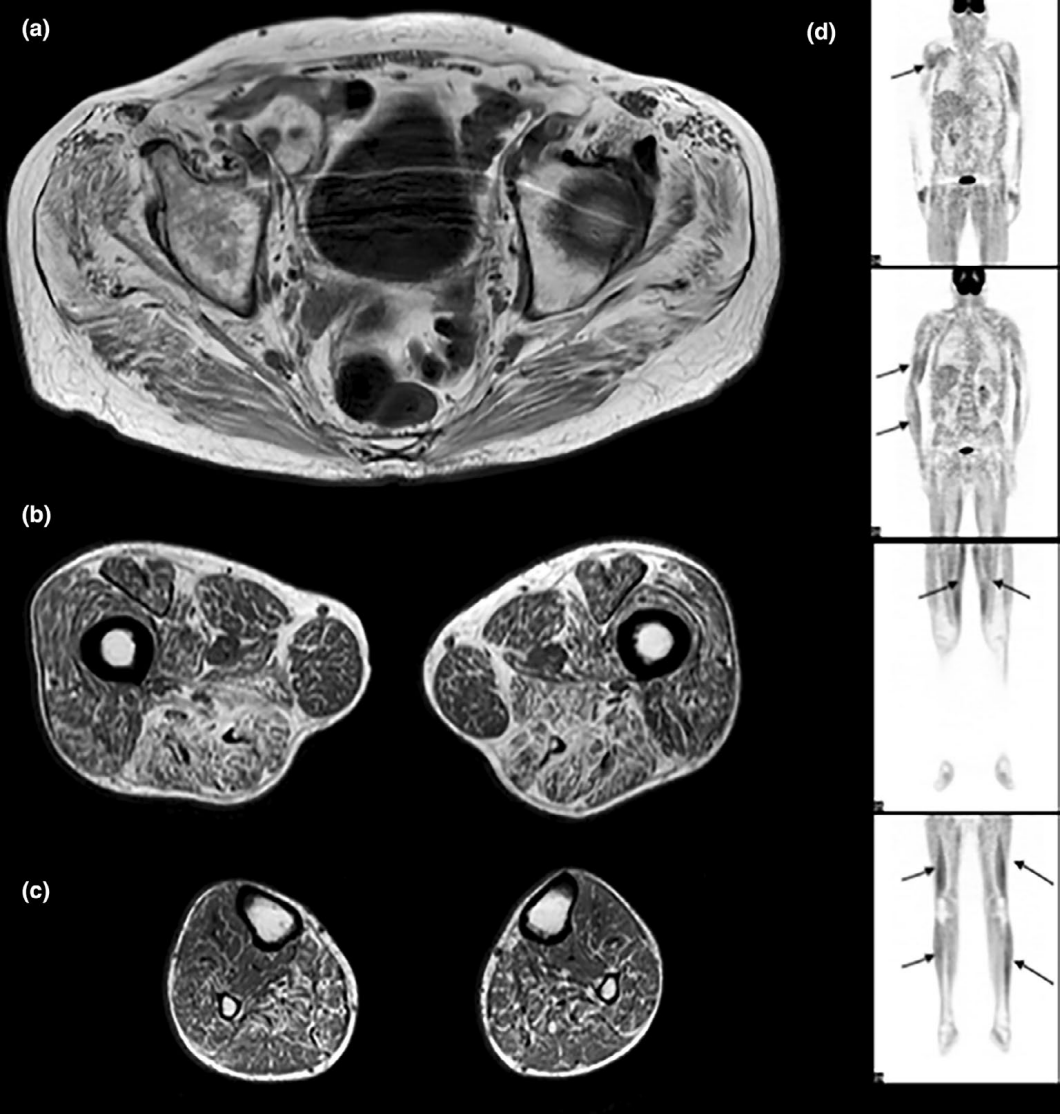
Muscle MRI and ^18^FDG‐PET scan studies in patient 3 at age 68. Muscle MRI of pelvic girdle (a) and thigh (b): fatty degeneration of gluteus, biceps femoris, semimembranosus, semitendinosus, biceps femoris, and adductors sparing gracilis. At the leg level (c), note fatty infiltration of the posterior compartment (soleus, gastrocnemius medialis, gastrocnemius lateralis) sparing tibialis posterior. ^18^FDG‐PET scan (d) shows increased symmetric FDG uptake in both proximal and distal muscles of all four limbs (arrows).
